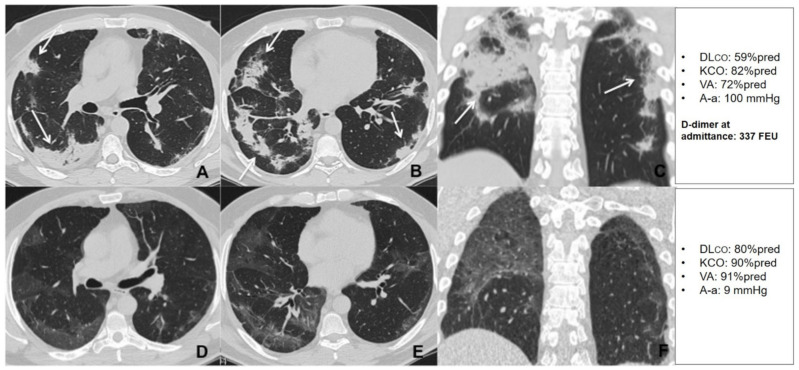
Unenhanced thin section axial (A,B) and coronal (C) CT images of the lungs obtained the 10th day post-admission in a 51-year-old man with no previous exposure to cigarette smoke, no cormorbidities, that was admitted to the high dependency respiratory care unit (HDRU) with severe respiratory failure (PaO_2_/FiO_2_ 182 mmHg) and treated with continuous positive airway pressure (CPAP) for 10 days. The images show peripheral consolidations (arrows) in both lungs, with predilection for posterior areas. Unenhanced thin section axial (D,E) and coronal (F) CT images of the same patient 6 weeks post-discharge show bilateral peripheral GGO with resolution of previous consolidations. The patient had a low D-dimer at admission and showed a significant improvement in his DLco during the recovery period.
